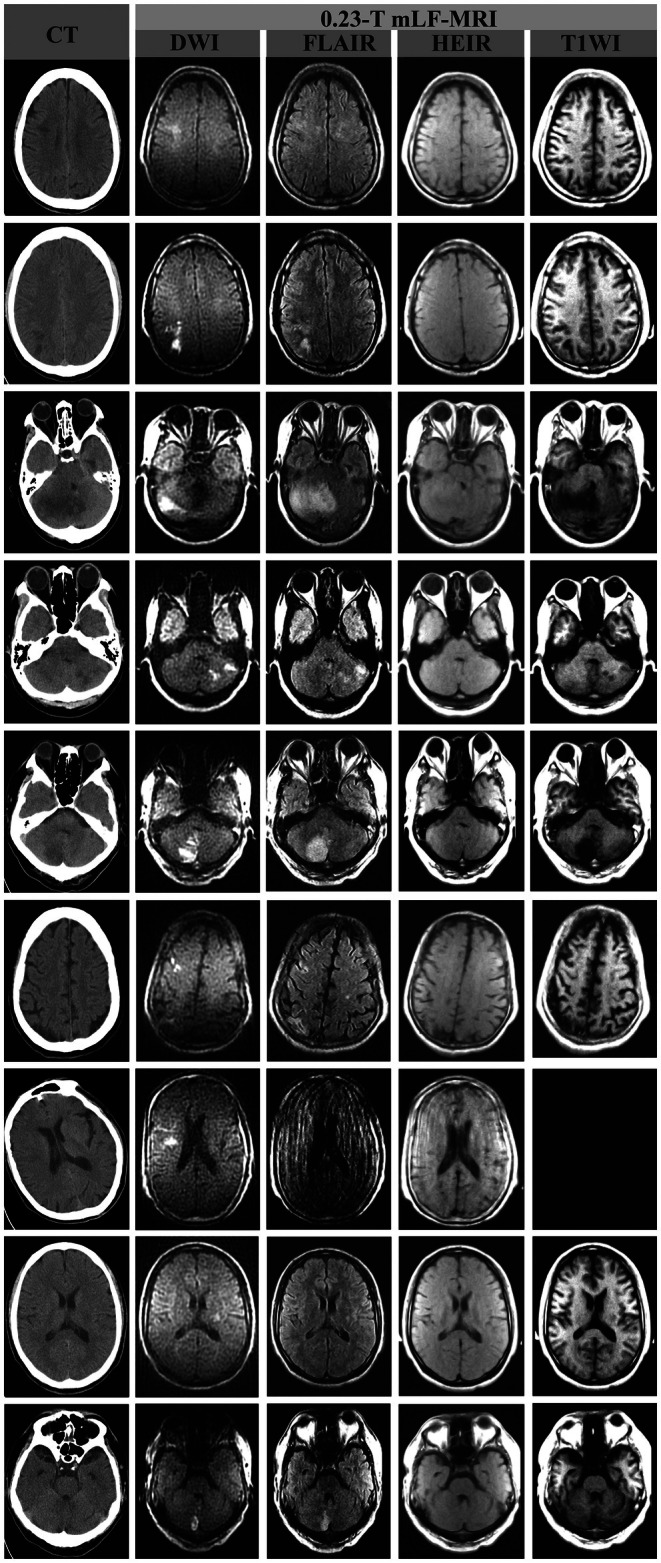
IS diagnosed in 9 patients presenting only with headache. CT, computed tomography; DWI, diffusion‐weighted imaging; FLAIR, fluid‐attenuated inversion recovery; HEIR, hematoma enhanced inversion recovery; IS, ischemic stroke; mLF‐MRI, mobile low‐field magnetic resonance imaging; T1WI, T1‐weighted imaging.
